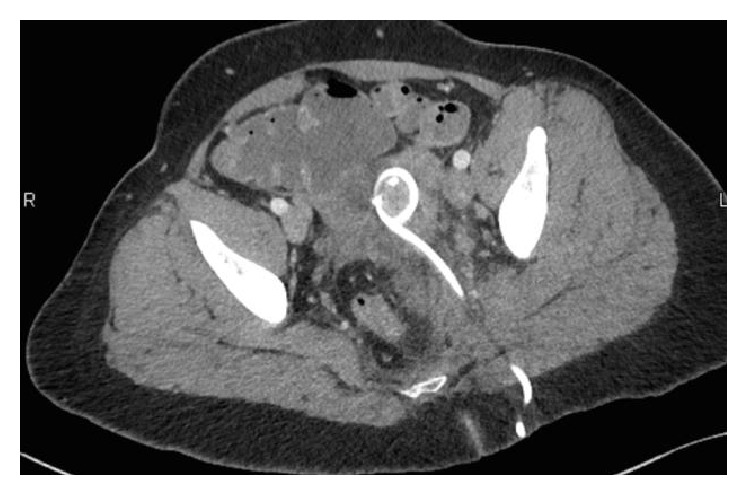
Ultrasound, fluoroscopic, and cone beam CT guidance; a 21-gauge needle was advanced into the fluid collection via a posterior transgluteal approach. A Greb set was used to secure access. An 8.5-French Cook all-purpose drainage catheter was placed over a wire into the collection and locked in place. Approximately 10 cc of purulent fluid was obtained and sent to the lab for analysis.
